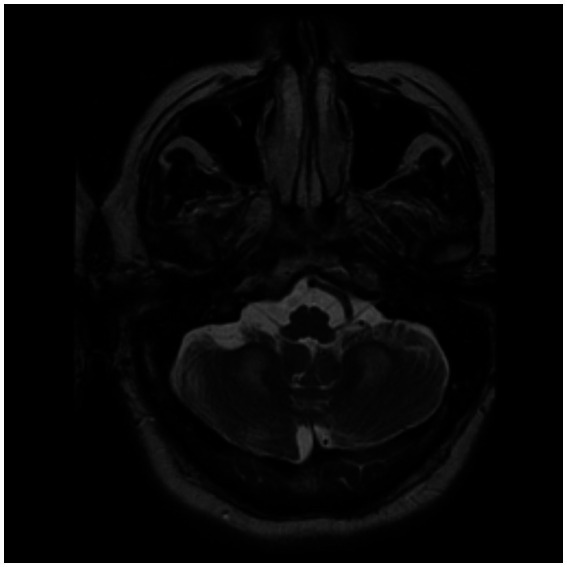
MRI of hyperintensities of the middle cerebellar peduncles (MCP).
